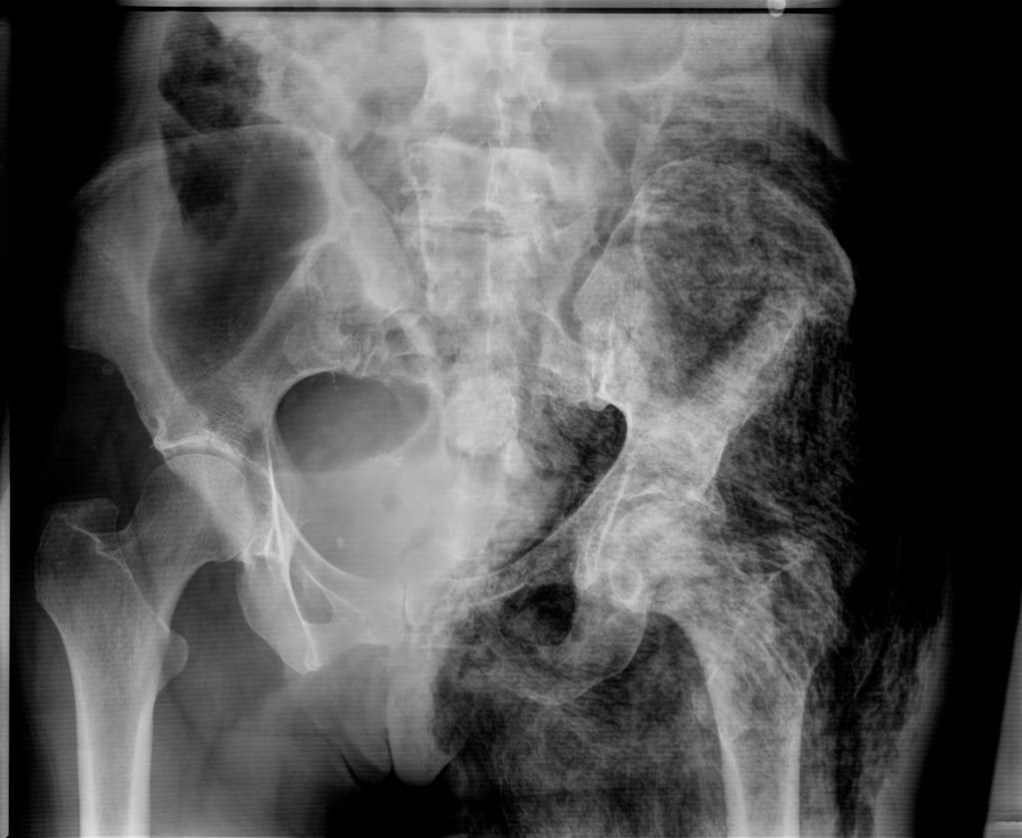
Anteroposterior pelvic X-ray highlighting diffuse linear lucencies of the left hemipelvis indicative of subcutaneous air along the fascial planes Courtesy of http://radiopaedia.org/cases/necrotising-fasciitis-1
